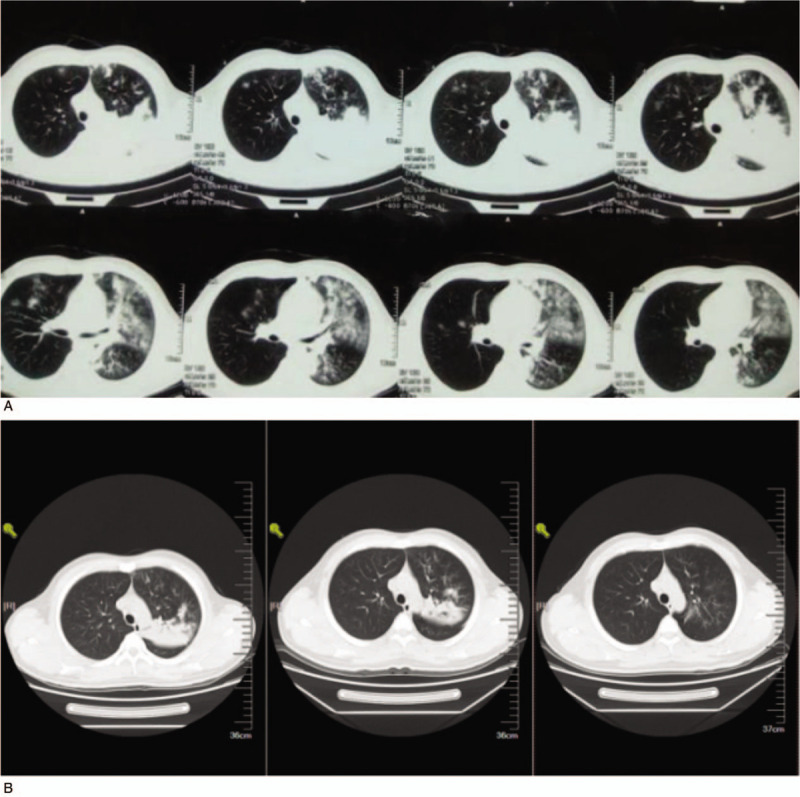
Chest computed tomography (CT) findings. (a) CT scan from May 2, 2017, showing consolidation in the left superior lobe and ground-glass opacification in both superior lobes. (b) CT scan from May 9, May 15, and June 20, showing gradual resolution of the consolidation in the left superior lobe and resolution of ground-glass opacification in both superior lobes.
